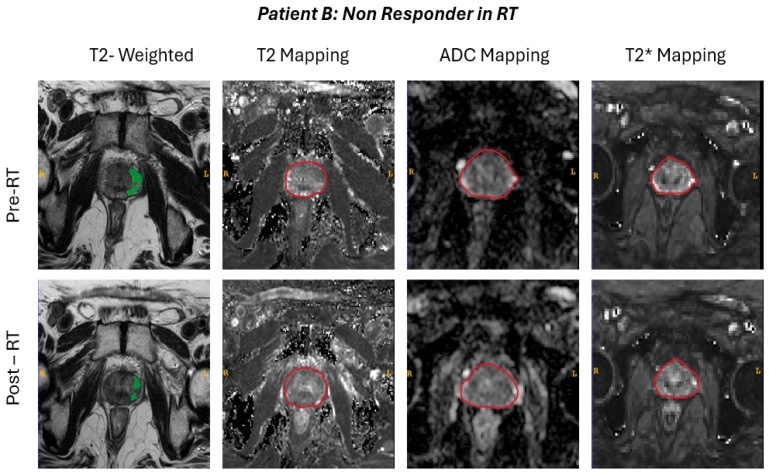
Multiparametric magnetic resonance imaging (mpMRI) of the prostate before and after radiotherapy in two patients. T2-weighted (T2w), T2 mapping, ADC mapping, and T2* mapping sequences are presented. In the upper panel (Patient A), post-RT changes consistent with therapeutic response are observed, including reduction in lesion size, increased ADC values, and signal normalization on T2w images and quantitative maps. In the lower panel (Patient B), no substantial response is evident, with persistently low ADC values, ongoing diffusion restriction, and absence of significant morphological or quantitative changes. Green markings indicate the lesions/region of interest (ROI) used for radiomics analysis, whereas red markings represent the whole-prostate segmentation used for mapping in this study. ADC: Apparent diffusion coefficient; RT: radiotherapy.
